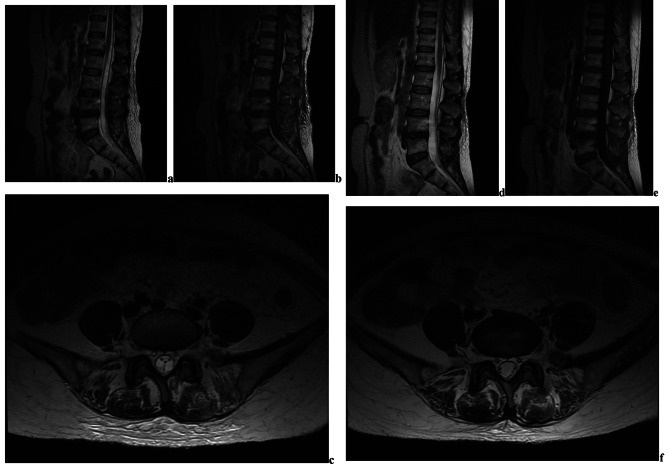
The characteristic MRI findings of SSH (the “Mercedes-Benz” sign). (a) postoperative T2-weighted sagittal MR image; (b) postoperative T1-weighted sagittal MR image; (c) postoperative T2-weighted axial MR image (the “Mercedes-Benz” sign); (d) 1 month follow-up T2-weighted sagittal MR image; (e) 1 month follow-up T1-weighted sagittal MR image; (f) 1 month follow-up T2-weighted axial MR image
